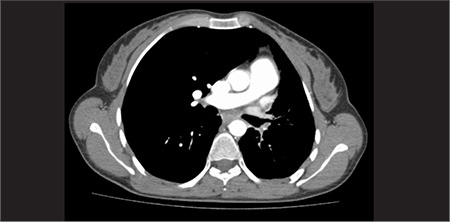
Axial oriented maximum-intensity-projection image from CT angiography shows small left hemithorax, mediastinal shift and absence of the left main pulmonary artery with left hilar bronchial artery collaterals
